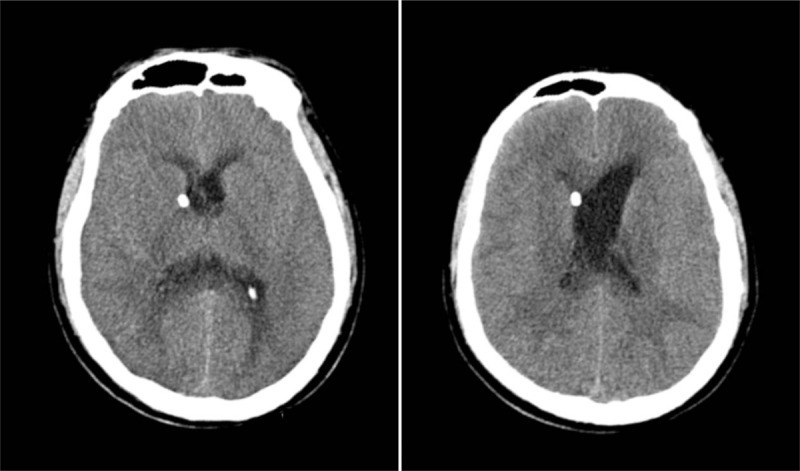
Head computed tomography showing cerebral edema and unshaped sulcus.
